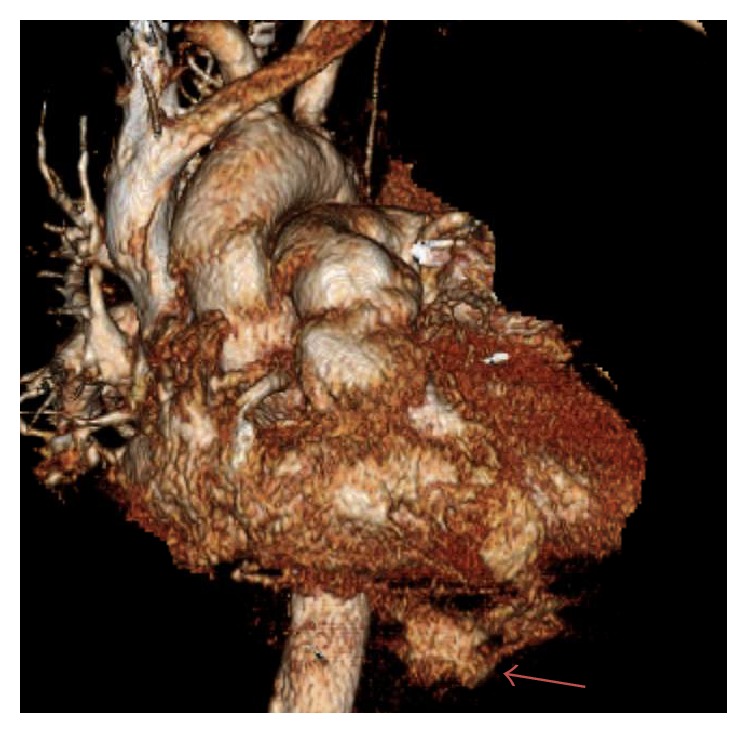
64-year-old male diagnosed with traumatic right ventricular rupture. 3D reformatted image from a routine contrast enhanced chest CT demonstrates contrast extravasation emanating from the right ventricle (arrow).
